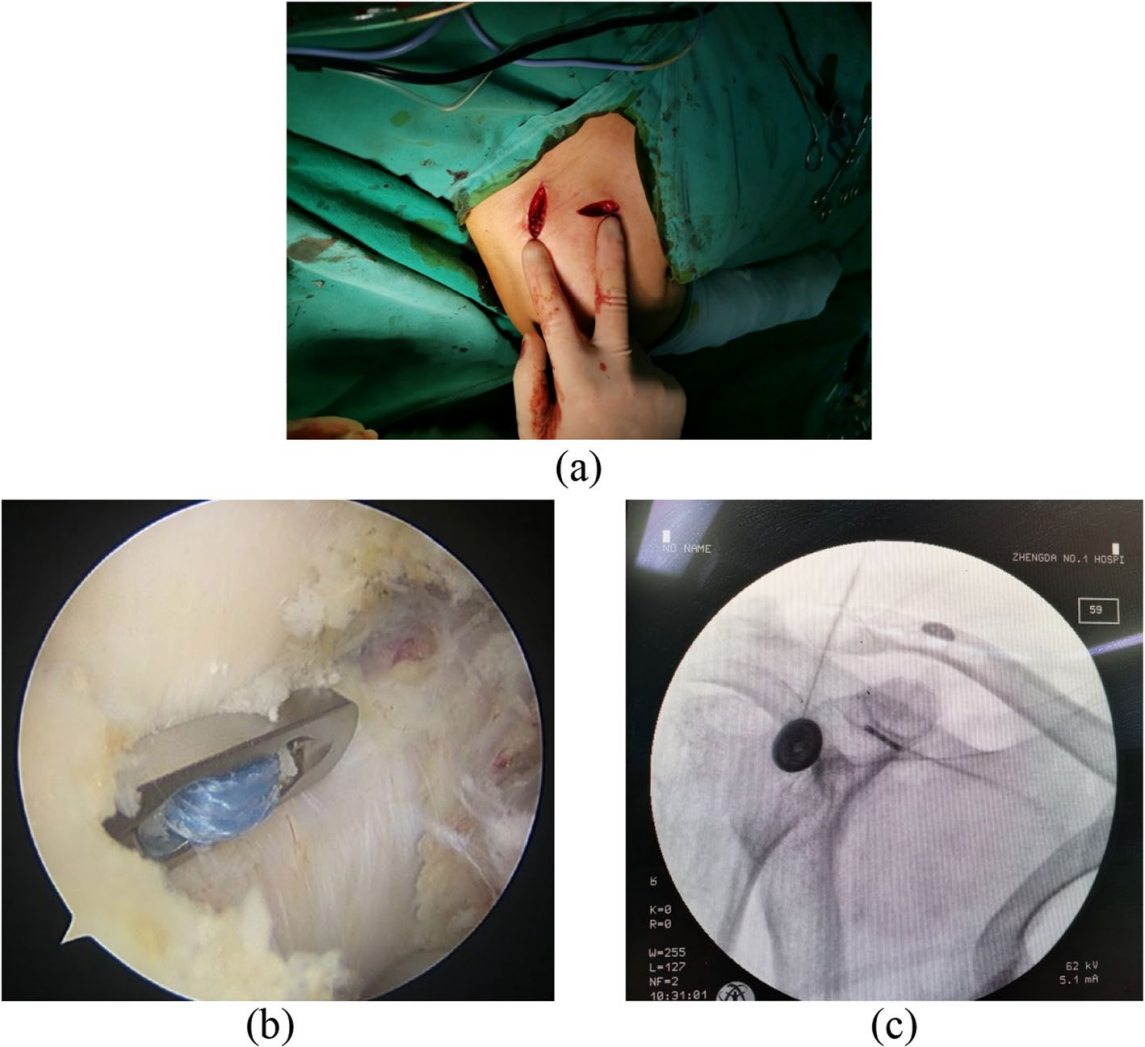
a: Arthroscopic surgical incision; b: the suspended titanium plate at the base of the coronoid process was observed under the arthroscope; c: the C-arm X-ray device showed good repositioning of the ACJ
